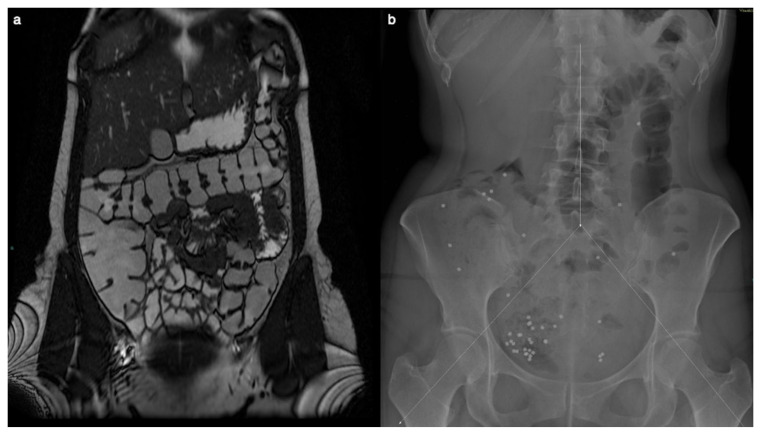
MR enterography showed no significant alteration two years before surgery, when the patient was already symptomatic. The Coronal T2-weighted image does not show signs of right colon amyloidosis (a). The plain abdominal X-ray is divided into three segments, and radiopaque markers are counted for each segment (b). Measurement of colonic transit time based on radio-opaque markers shows delayed colonic transit time (39/60 markers). The distribution of the markers, with a majority in the right region (31/39), suggests reduced motility at the level of the cecum and ascending colon.
